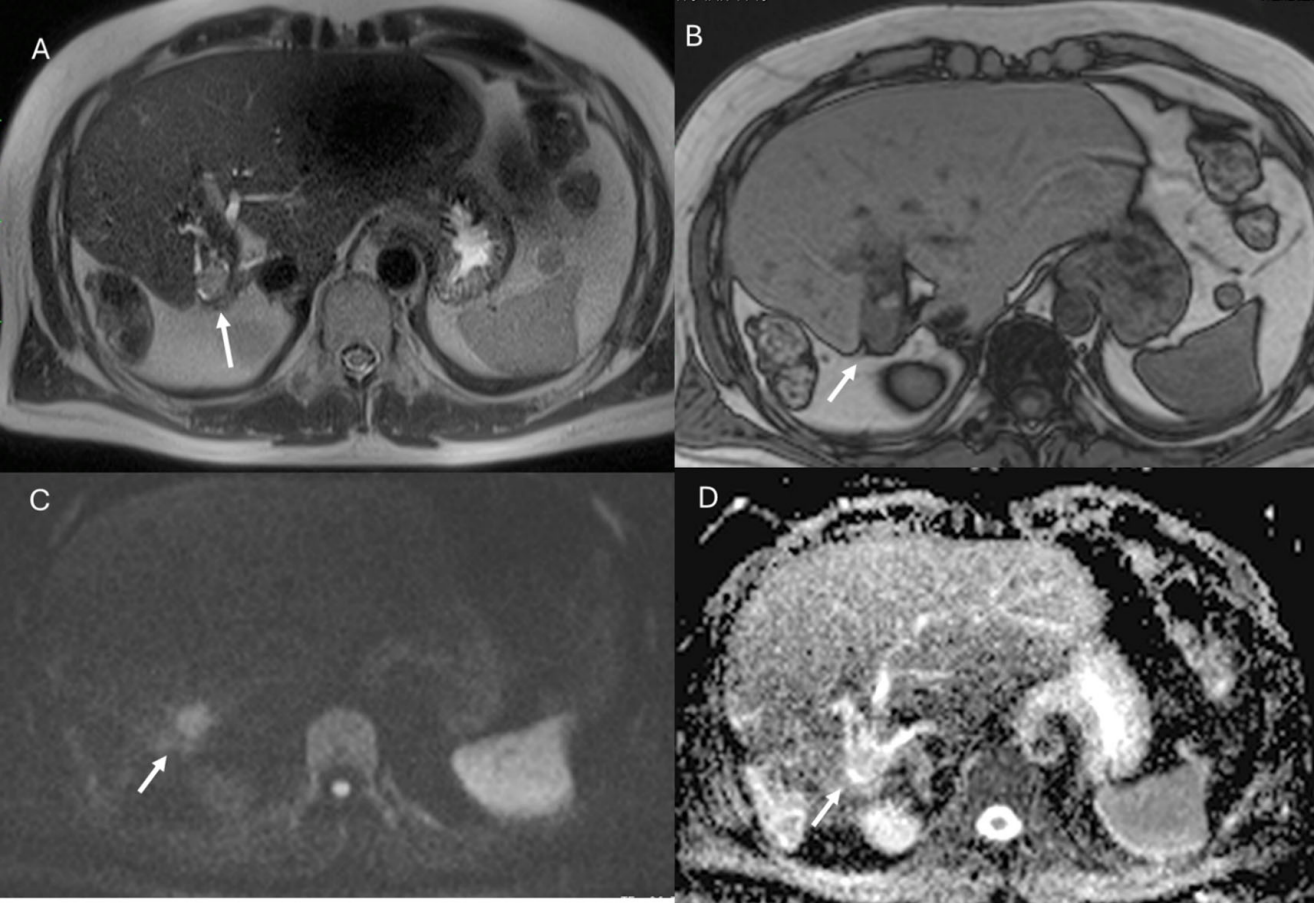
MRI assessment of residual disease after surgical resection. The lesion shows similar features to the patient in Figure 9, with hyperintense signal in T2-W sequence (A), hypointense signal in T1-W out-of-phase sequence (B), restricted diffusion on b = 800 s/mm² (C), and hypointense signal on ADC map (D). In this case, DWI is suggestive of residual lesion.
